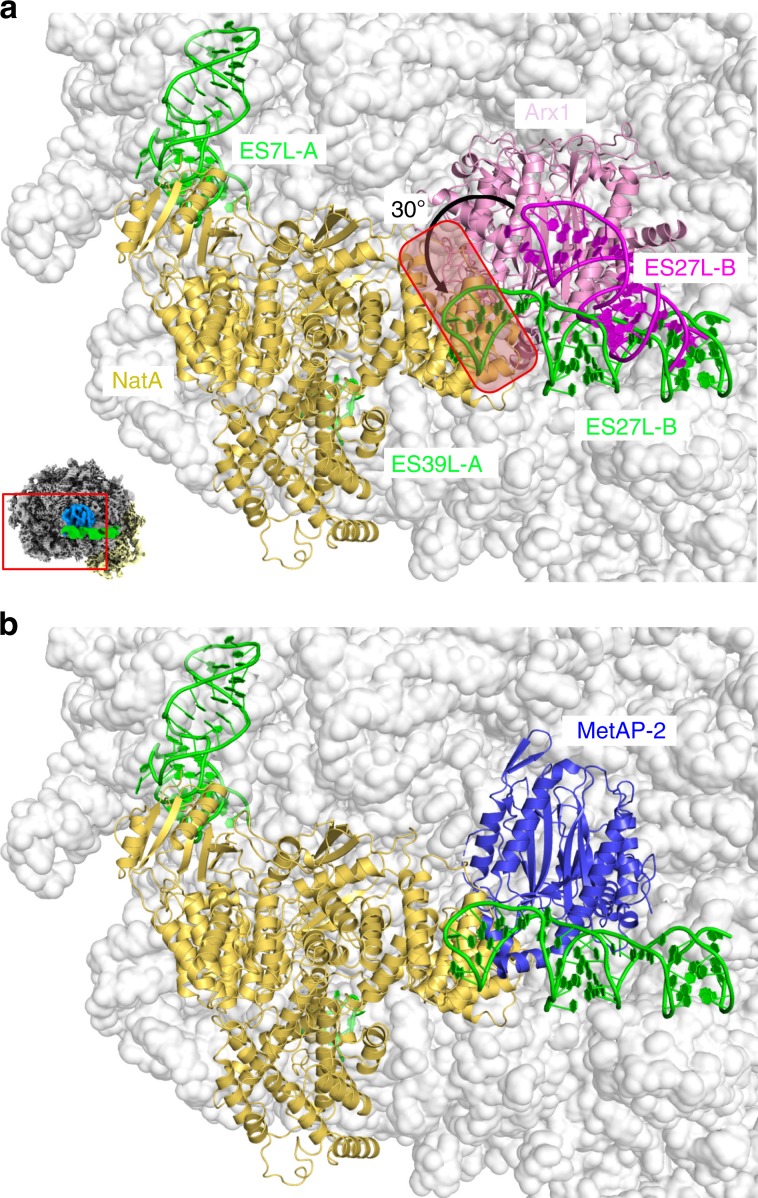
PA2G4 proteins as MetAP-2 structural homologs. a Structure of the NatA–ribosome complex from yeast^25^ (PDB ID: 6HD7) superposed with Arx1–ES27L (in pink tones) taken from the Arx1–ribosome complex^14^ (PDB ID: 5APN). ES27L-B was built de novo in this study. The C-terminal region of Arx1 clashes (boxed region) with the Naa10 subunit, and ES27L-B conformation is different (arrow). The view is identical to Fig. 1c top panel, and as indicated by the small representation in the corner. b Proposed model for simultaneous MetAP-2/NatA binding in eukaryotic co-translational protein modification based on the yeast NatA complex and the human MetAP-2 X-ray structure^54^ (PDB ID: 1BN5) superposed on Arx1.
